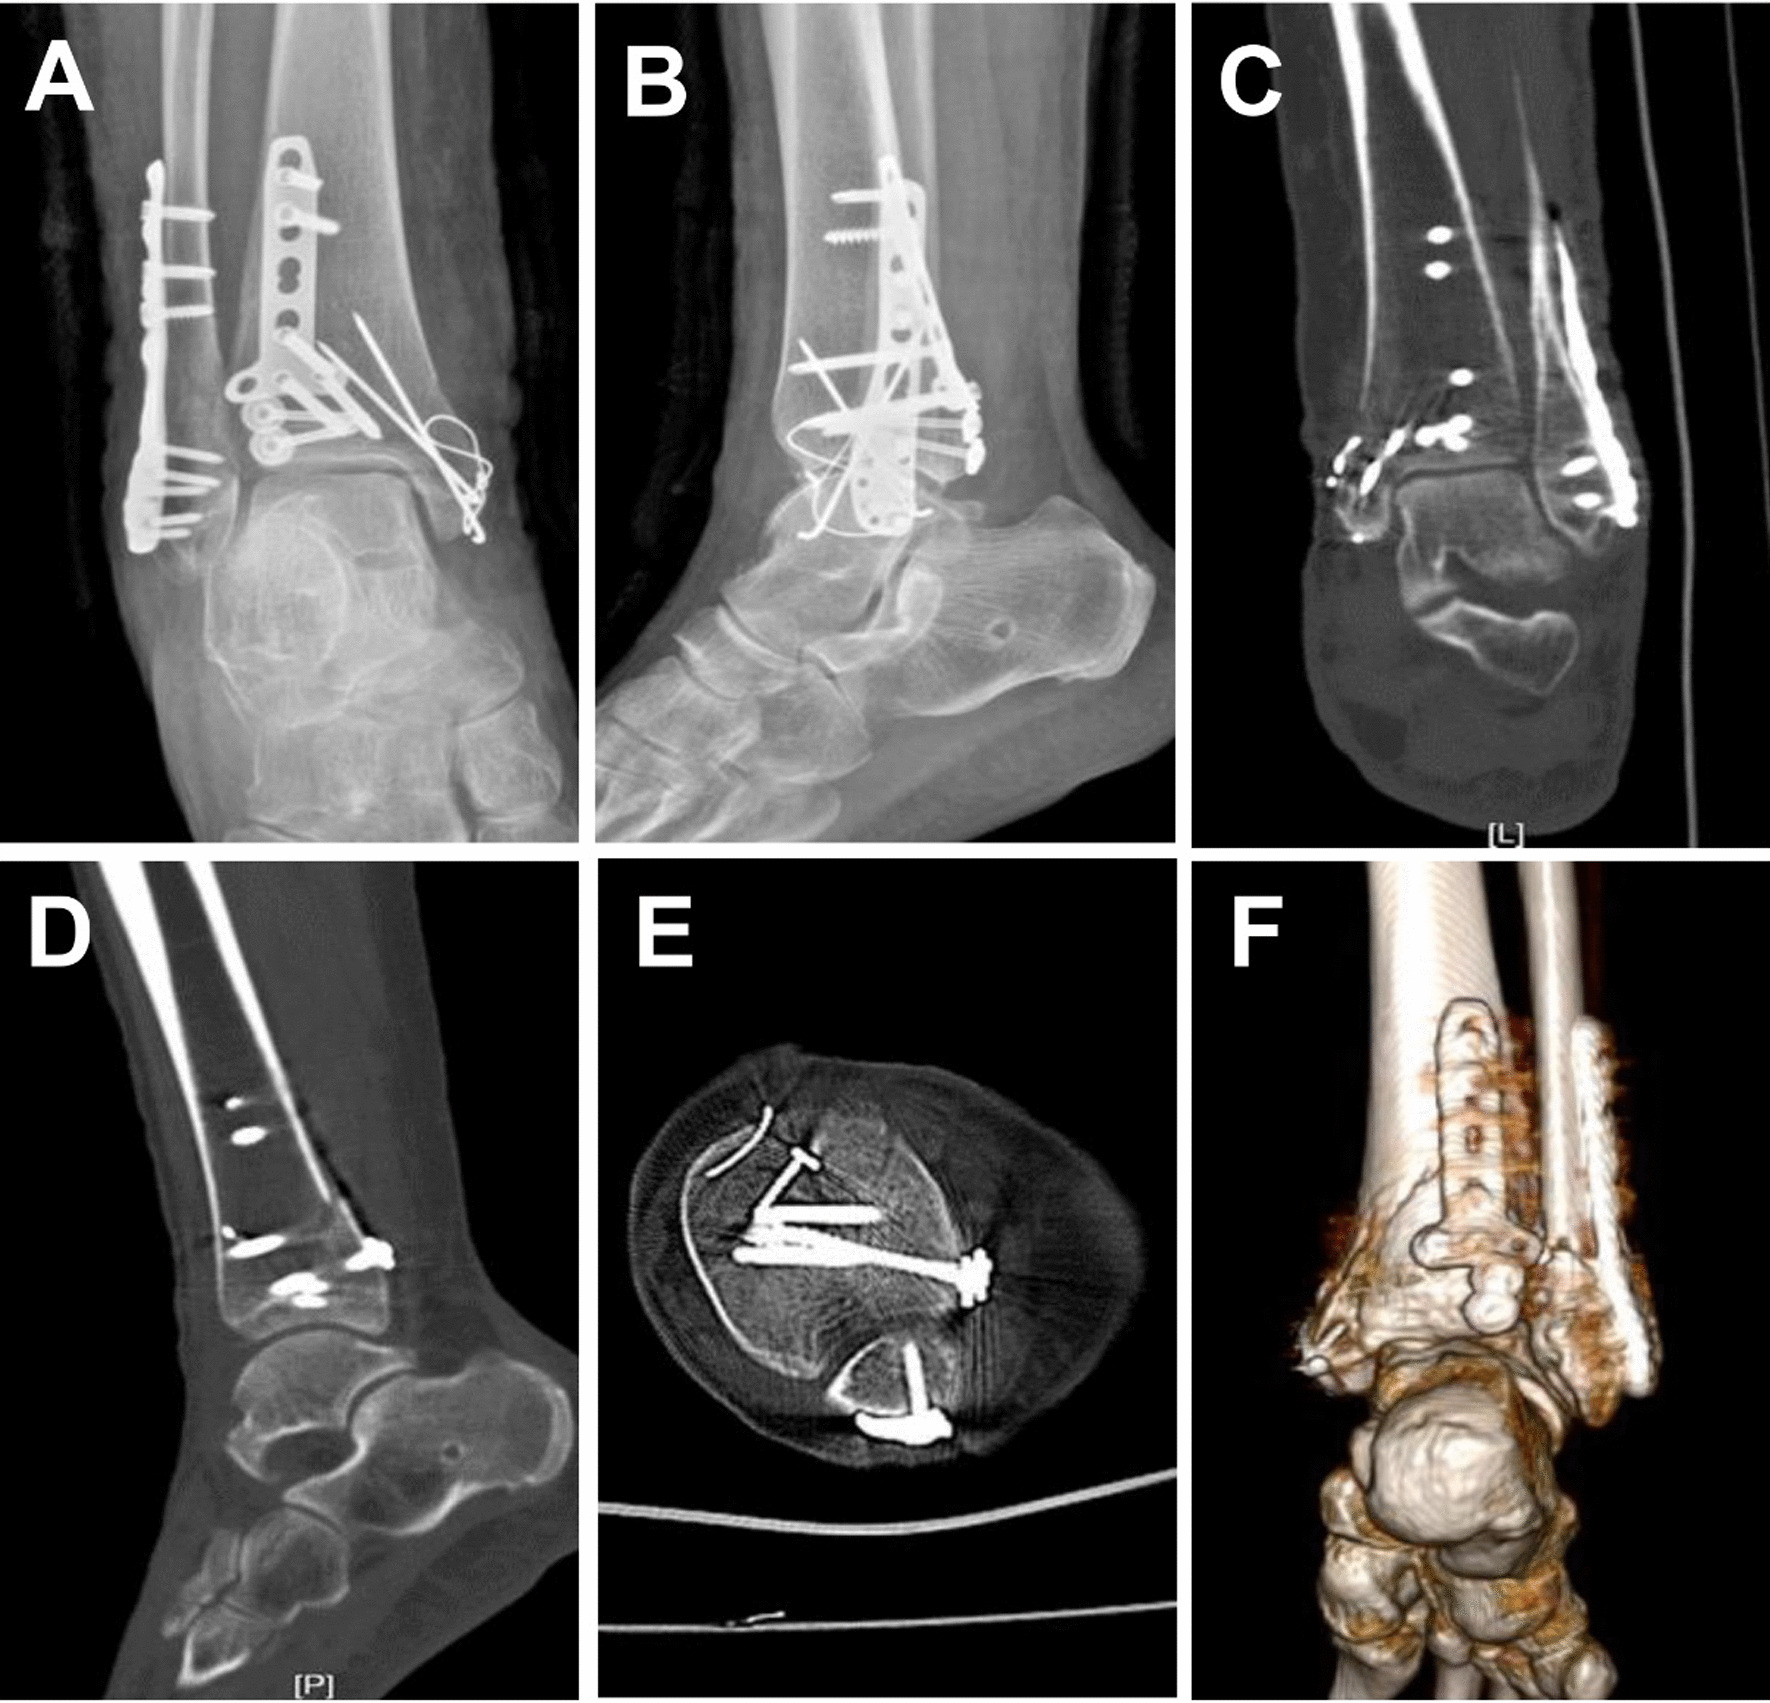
The patient’s postoperative X-ray and CT 3D reconstruction showed anatomic reduction in the fracture. A, B X-ray in anterior–posterior and right-left positions. C CT coronal plane. D The sagittal subluxation and step sign were corrected. E CT transverse views. F Representative image of CT 3D reconstruction
